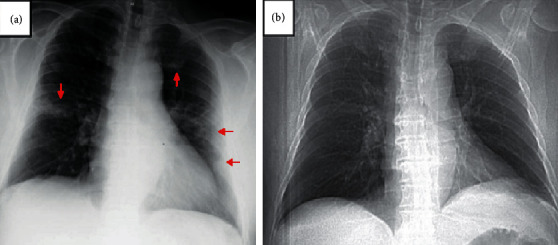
Chest radiographs of the patient. (a) Chest radiograph on presentation to the emergency department; the arrows delineate areas of patchy opacities that tend to favor the lung periphery. (b) Scout film from a previous high-resolution chest CT approximately 1.5 years prior to his COVID-19 presentation for comparison.
